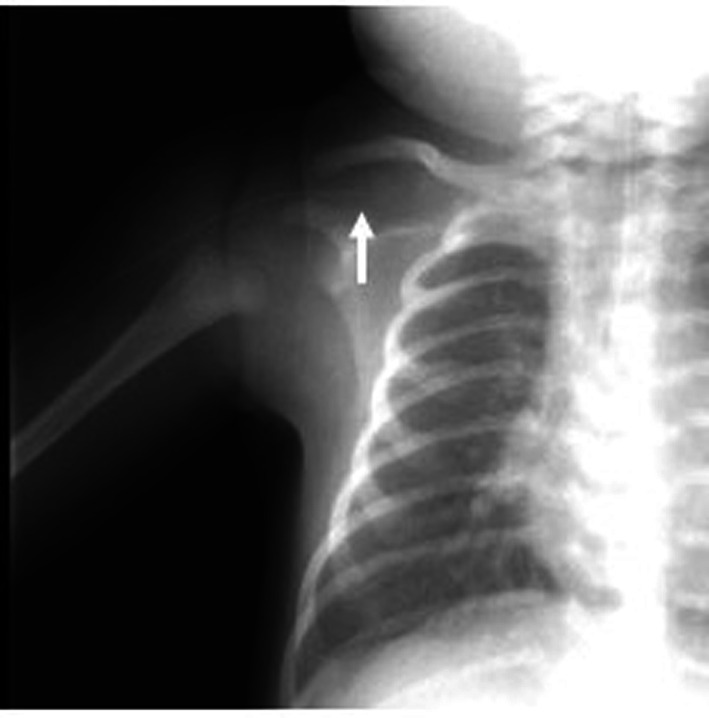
Chest X-ray taken on the day of PICC insertion. Arrow indicates the tip of the catheter. The tip is located in the right subclavian vein
